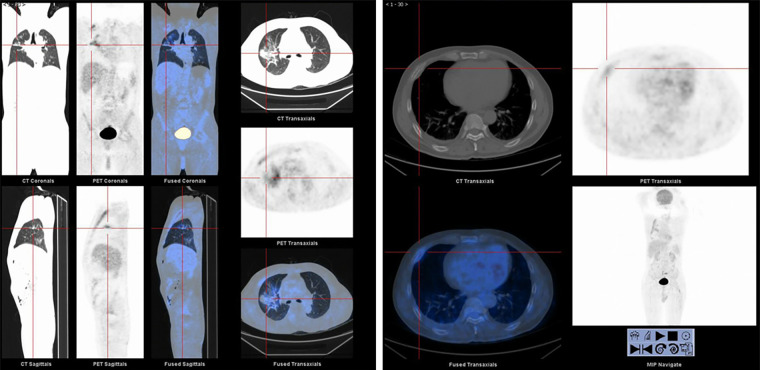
PET-CT images of primary mucinous lung adenocarcinoma with rib metastases.
